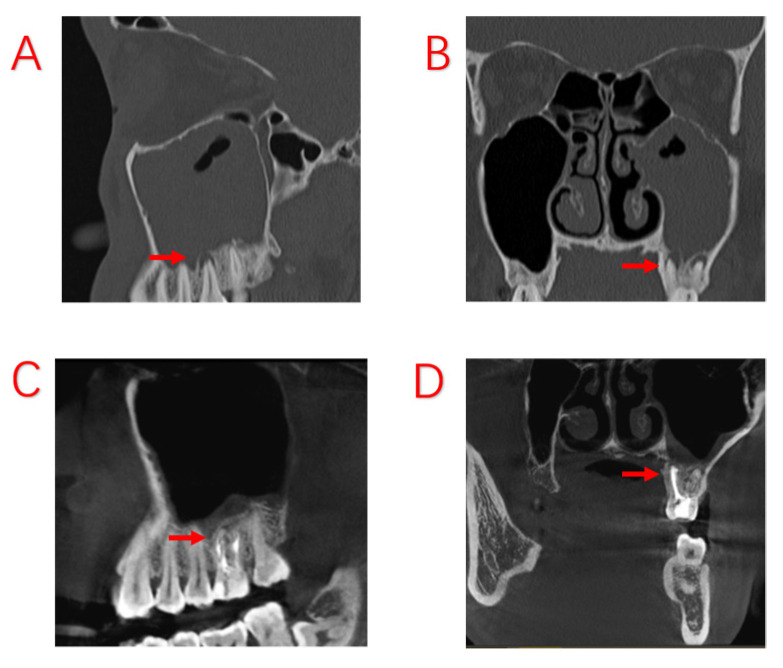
Odontogenic sinusitis due to periapical inflammation. Symptoms disappeared after root canal treatment; no ESS was performed. (A,B): Before root canal treatment, the patient developed a purulent discharge with a foul odor and obstruction of the maxillary sinus opening. (C,D): After root canal treatment, infection of the dental pulp was controlled and the symptoms of sinusitis had disappeared, with the mucosa of the maxillary sinus returning to normal. Red arrows indicate sites of apical inflammation.
